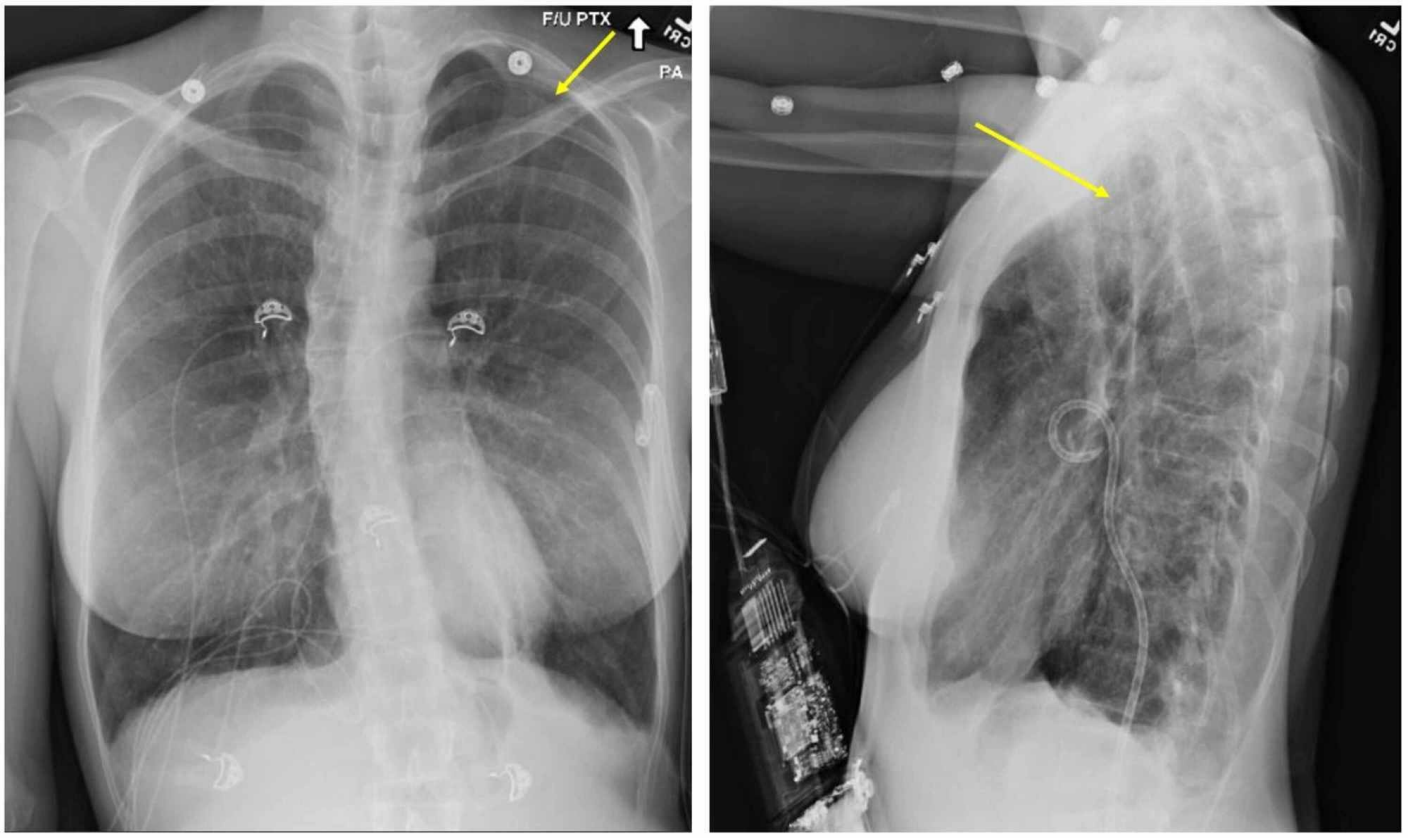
X-ray chest PA and lateral Left-sided pneumothorax PA: posteroanterior
